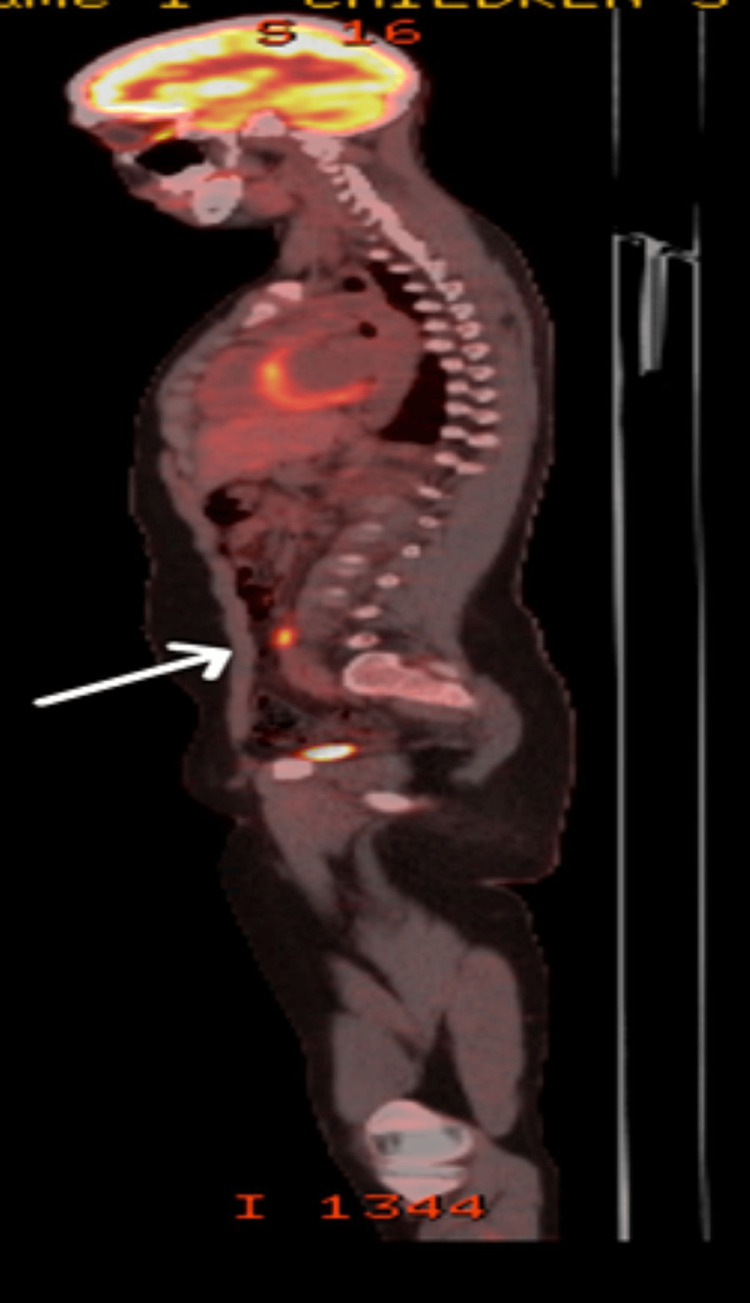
Axial fused 18F-FDG PET/CT image obtained four weeks postoperatively demonstrating focal hypermetabolic uptake within a nodular lesion adjacent to the right psoas muscle (arrow), initially concerning for residual or recurrent Burkitt lymphoma.
